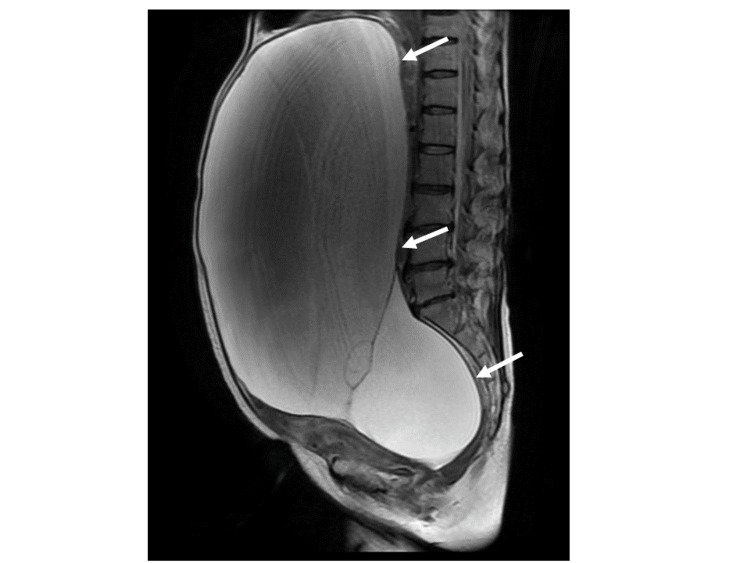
Ovarian cyst MRI (T2-weighted imaging) demonstrates a huge pelviabdominal cyst measuring 37 cm without solid components within the cyst (arrows).
